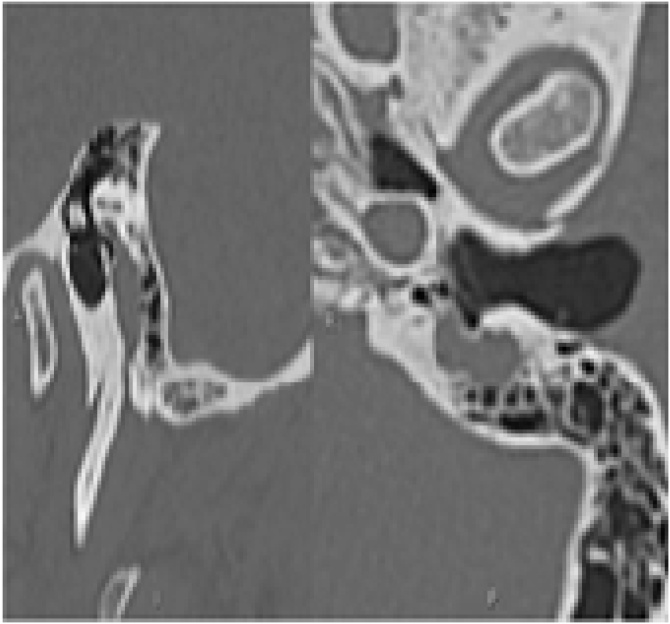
Pre-2nd surgery, temporal bone CT scan (sagittal and axial) showing an expansive lesion in the Fallopian canal, limited to the mastoid portion of the nerve.
